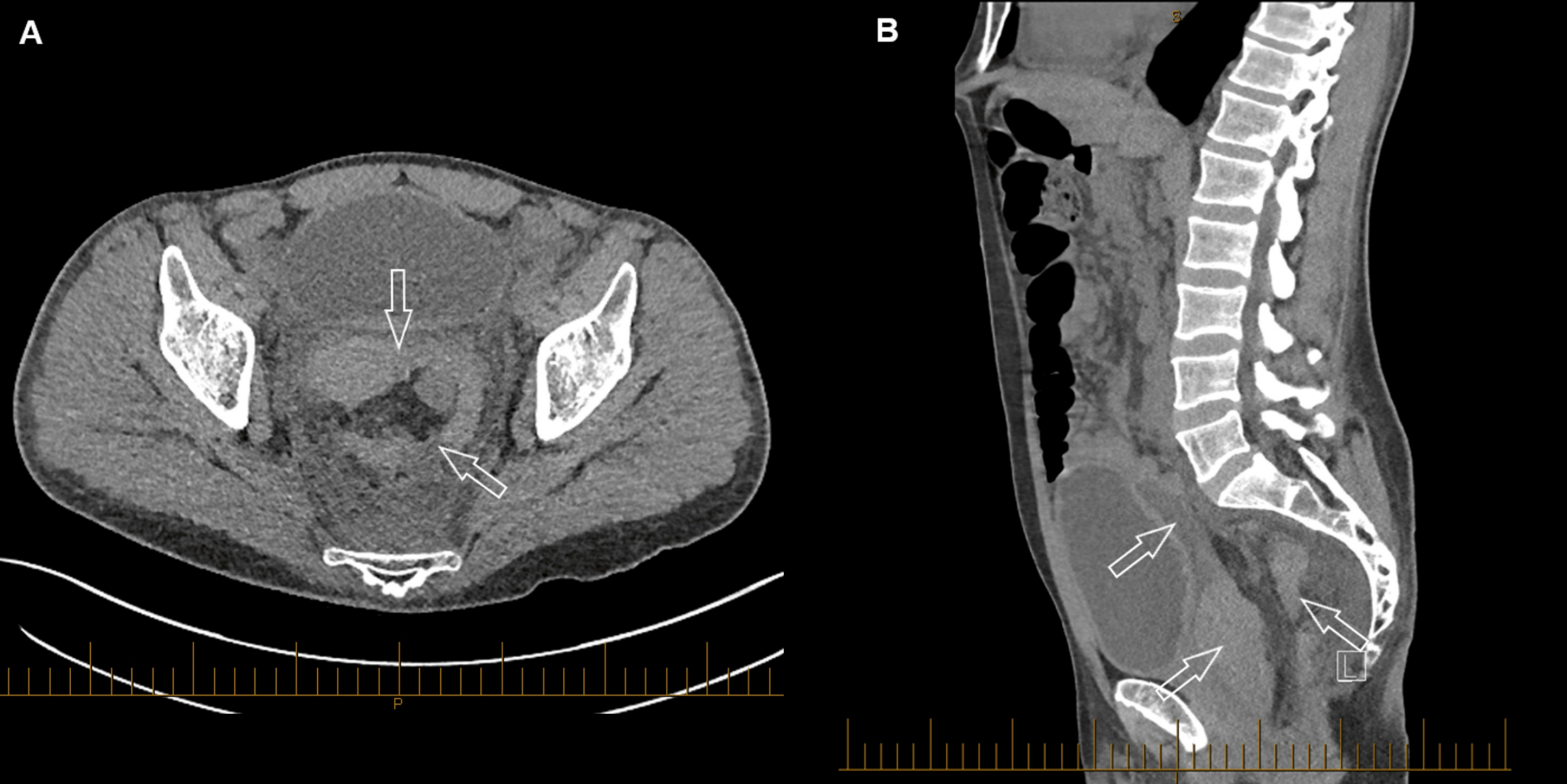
CT of the abdomen and pelvis two days after the Altemeier procedure. (A) axial, (B) sagittal, demonstrating a hyperdense lesion consistent with postoperative haematoma in the rectovesical pouch and extending to the presacral space. A slightly hyperdense haemoperitoneum in the pelvis is noted in (B).
